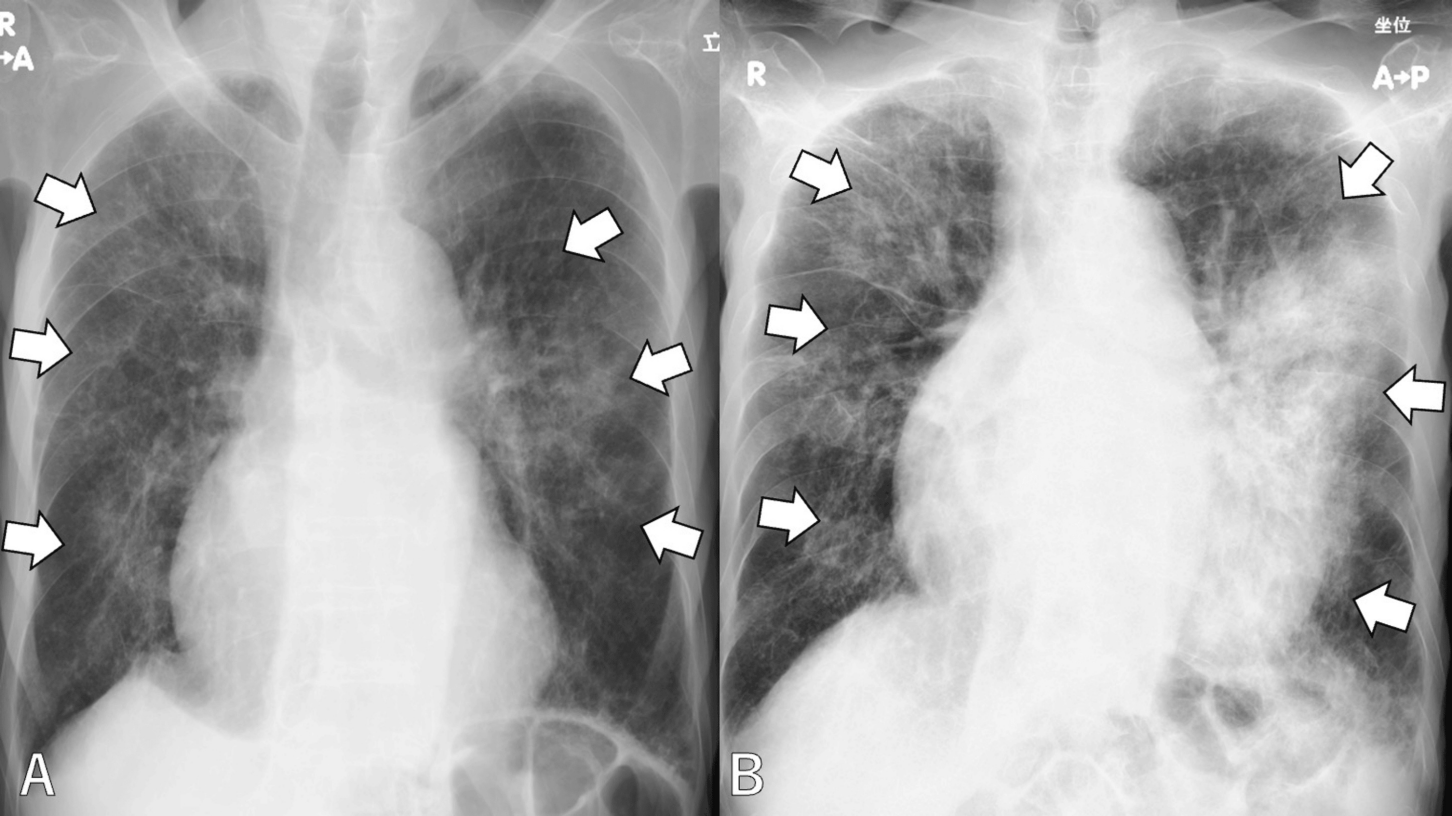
Chest radiography showing worsening consolidation in the left mid-lung field (B) compared with the previous imaging at discharge (A), as well as a new ground-glass opacity in the right middle-to-lower lung zones (white arrows)
